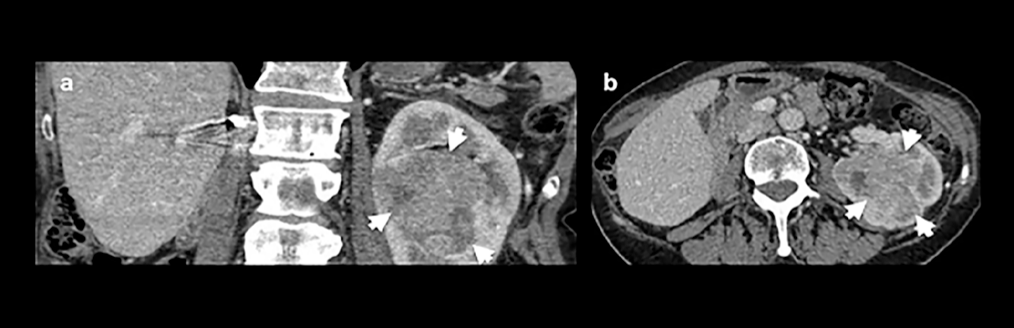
Contrast-enhanced CT of the abdomen at the level of left kidney. (a) Coronal and (b) axial slices showing an incidental finding of a 6.8 × 5.1 × 6.1 cm mass in the left kidney (arrows). There were no radiological signs of metastatic disease on CT of chest, abdomen and pelvis.
